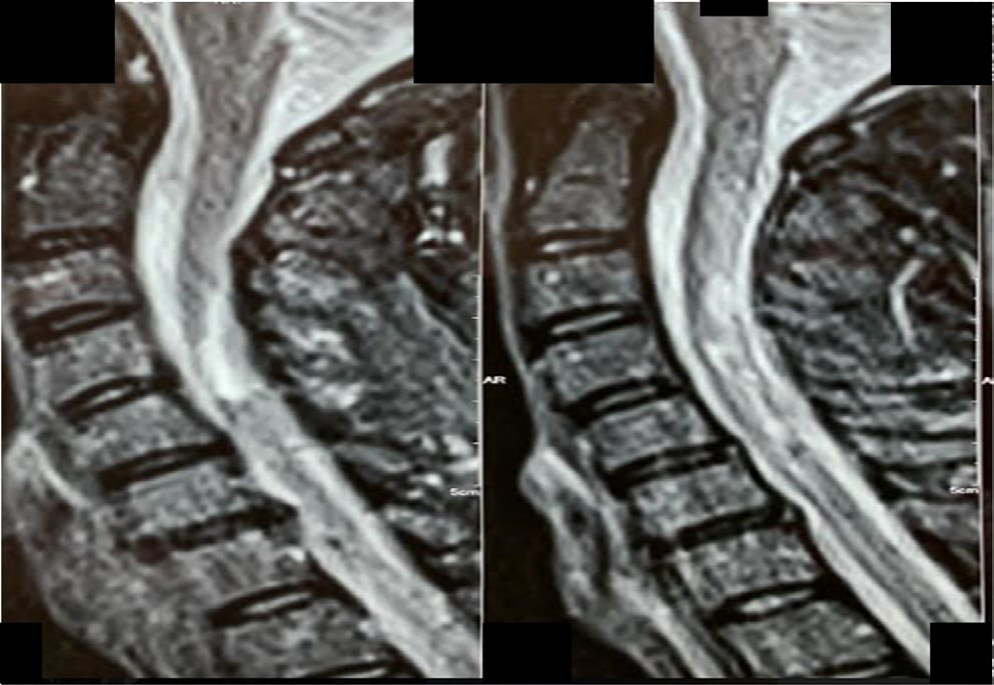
Fluid‐attenuated inversion recovery (FLAIR) cervical spine MRI of the patient revealing a hyperintense lesion at the level of C3‐C4
